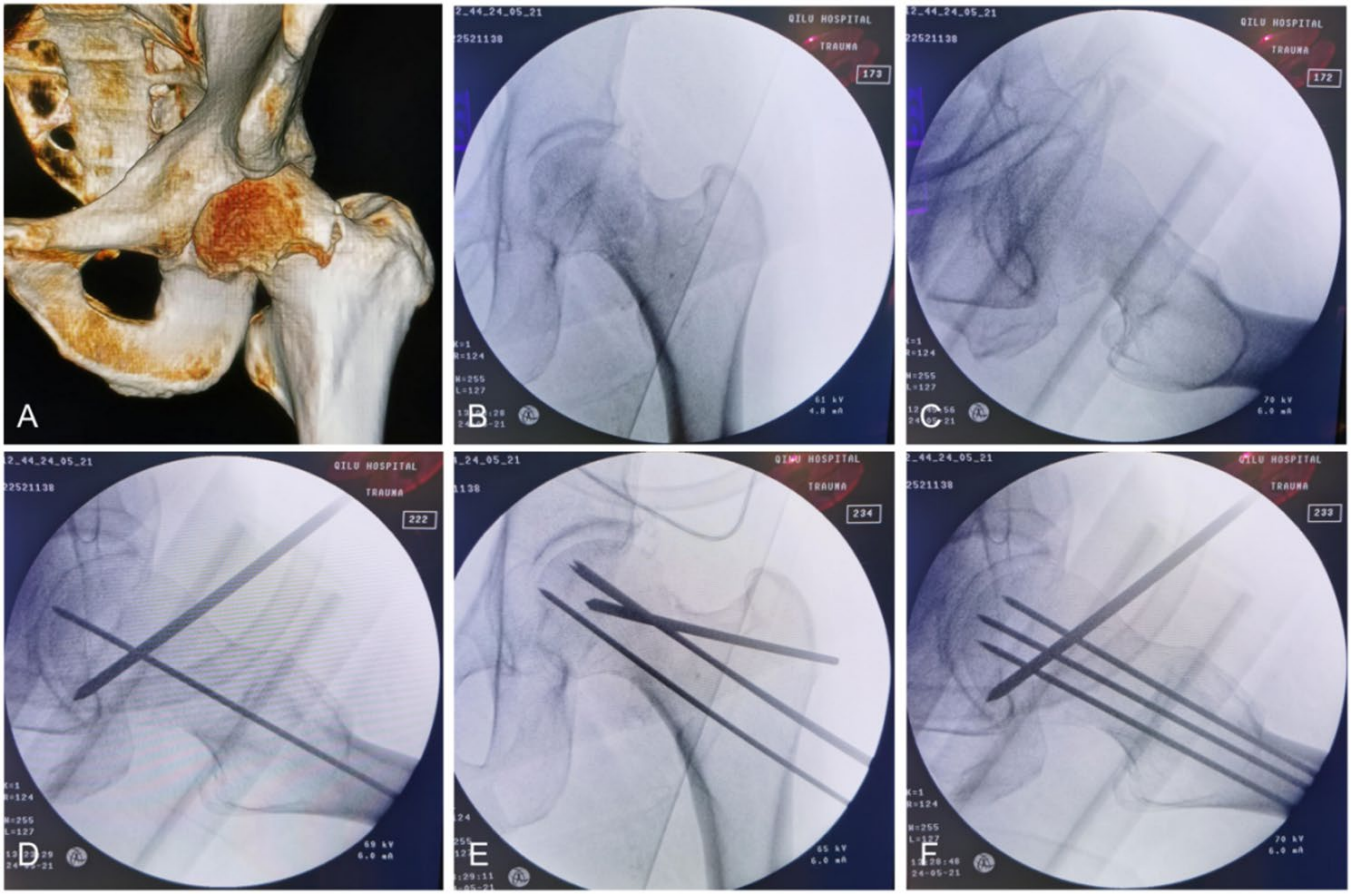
A 45-year-old male with a left femoral neck fracture (Garden III, Pauwels II). A Preoperative CT scan. B, C Despite traction, adduction, and internal rotation, significant posterior tilt of the femoral head persists. D Reduction was achieved by levering the femoral head anteriorly using a Steinmann pin and Kirschner wires. E, F Three guide pins were placed in an inverted triangular configuration
